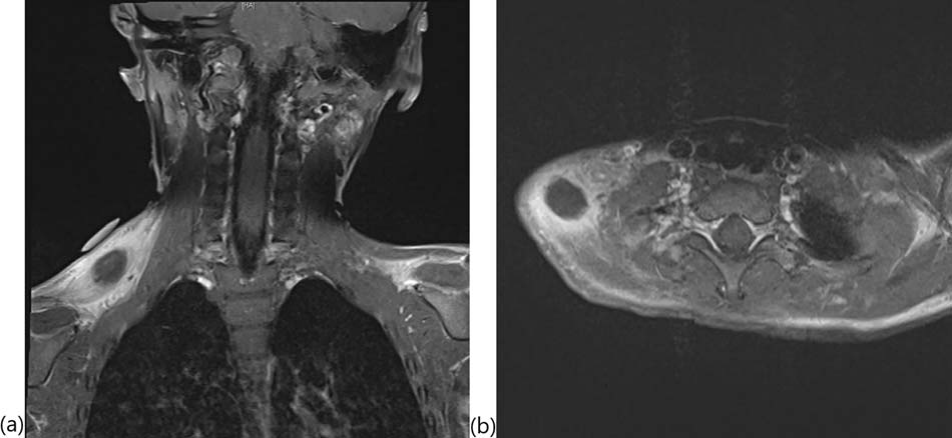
T1-weighted MRI with fat saturation showing the intramuscular mass in the (a) coronal and (b) axial planes.
